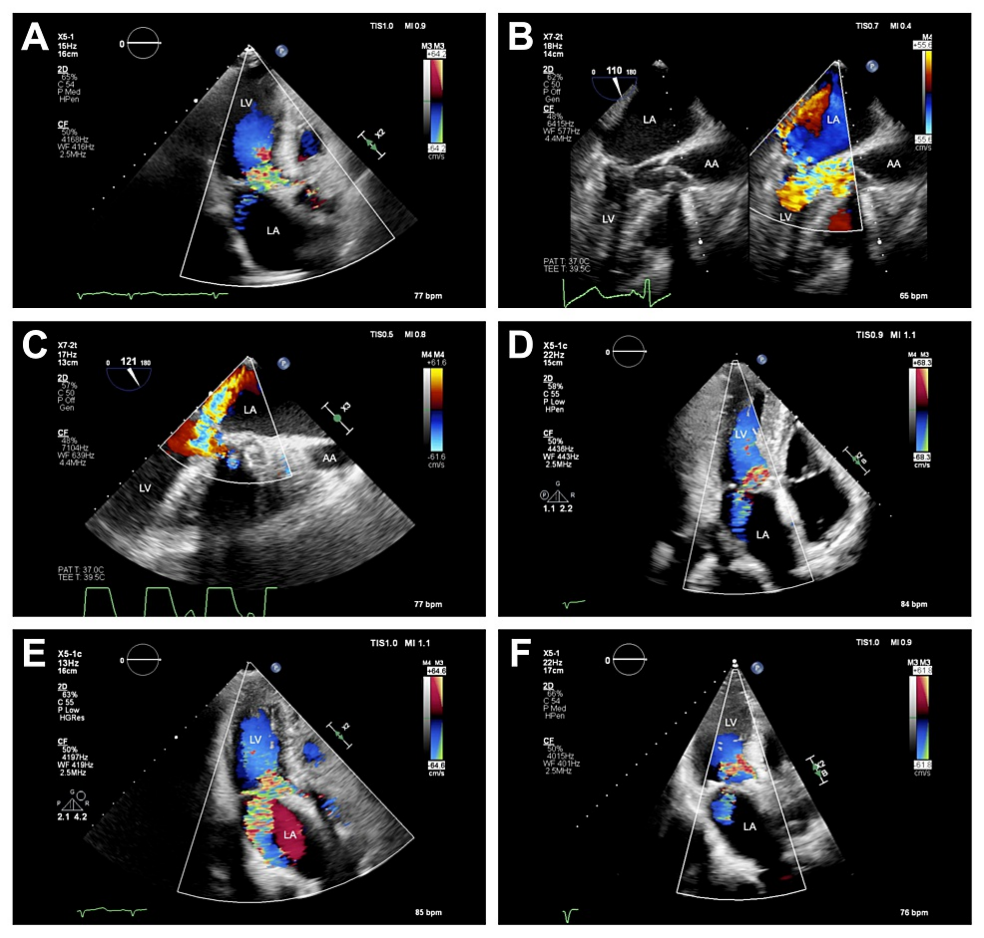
Echocardiography (A) A color Doppler image obtained in an apical long-axis view of preoperative transthoracic echocardiography shows mitral regurgitation secondary to systolic anterior motion of the mitral valve. (B) An image obtained in a mid-esophageal long-axis view using color compare imaging of intraoperative transesophageal echocardiography before implantation of a transcatheter aortic valve shows mitral regurgitation with an eccentric jet directed posteriorly to the atrial roof combined with systolic anterior motion of the mitral valve. A B-mode image is shown on the left and a color Doppler is shown on the right. (C) A color Doppler image obtained in a mid-esophageal long-axis view of intraoperative transesophageal echocardiography immediately after implantation of a transcatheter aortic valve shows apparent exacerbation of mitral regurgitation with a centrally directed jet. (D) A color Doppler image obtained in an apical long-axis view of transthoracic echocardiography after admission to the intensive care unit shows mitral regurgitation with vena contracta width of 0.46 cm and jet area to left atrial area ratio of 29%. (E) A color Doppler image obtained in an apical long-axis view of transthoracic echocardiography after the termination of the administration of landiolol and phenylephrine on postoperative day 1 shows exacerbation of mitral regurgitation with vena contracta width of 0.60 cm and jet area to left atrium area ratio of 33%. (F) A color Doppler image obtained in an apical long-axis view of transthoracic echocardiography after re-administration of landiolol and cibenzoline on postoperative day 3 shows improvement of mitral regurgitation with vena contracta width of 0.48 cm and jet area to left atrium area ratio of 27%. AA, ascending aorta; LA, left atrium; LV, left ventricle
